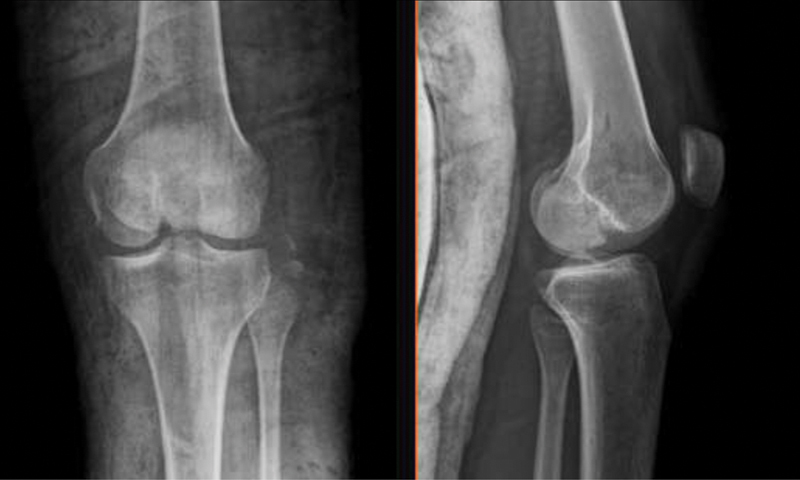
Anteroposterior and lateral view of radiographs.
